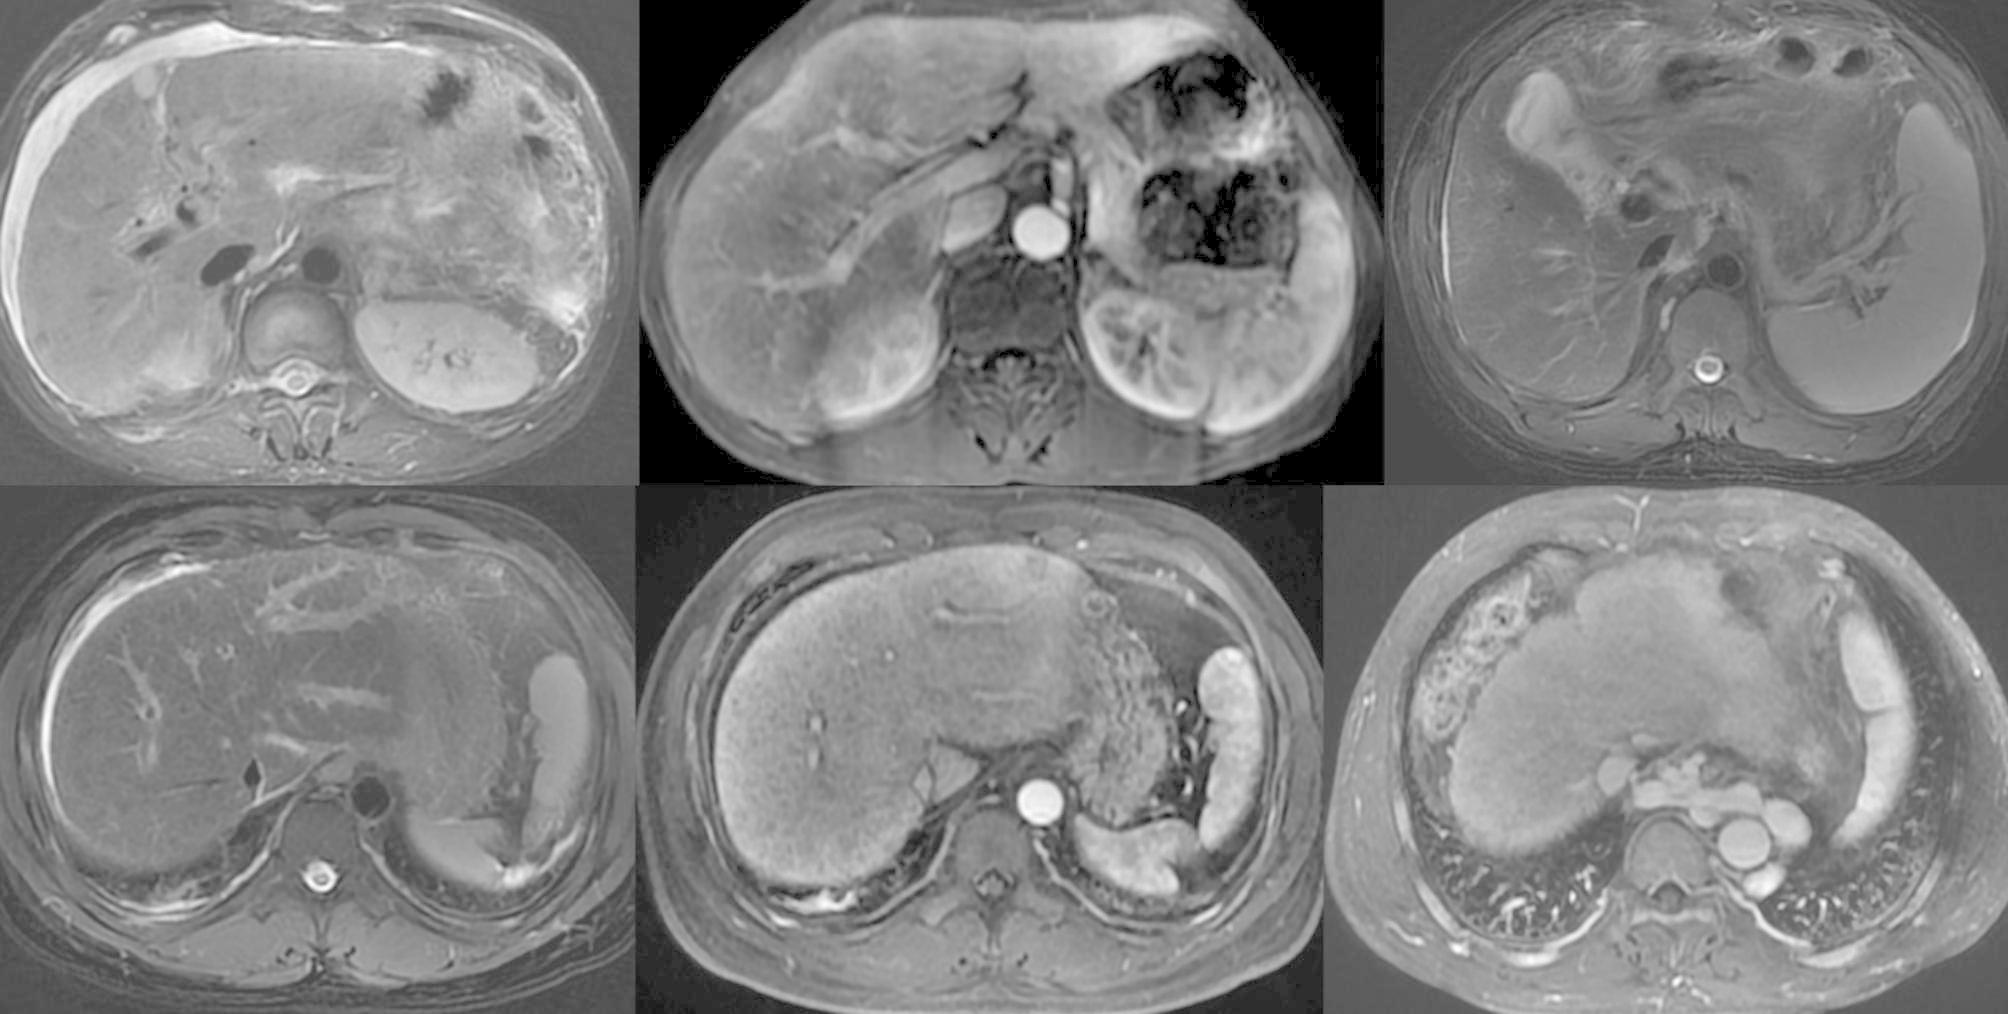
MR imaging features of ACLF. (a) Diffuse hyperintense of the liver on T2WI and ascites in patients with decompensated cirrhosis; (b) patchy enhancement of the liver mainly located in the left lobe at the arterial phase in patients with chronic hepatitis B; (c) gallbladder wall thickening on T2WI in patients with compensated cirrhosis; (d) and (e) periportal edema, (d) hyperintense tramlines or rings around the flow void in the segmental portal vein in patients with chronic hepatitis B on T2WI and (e) low intensity at corresponding locations at the PVP; and (f) gastric varix in patients with decompensated cirrhosis at the PVP.
